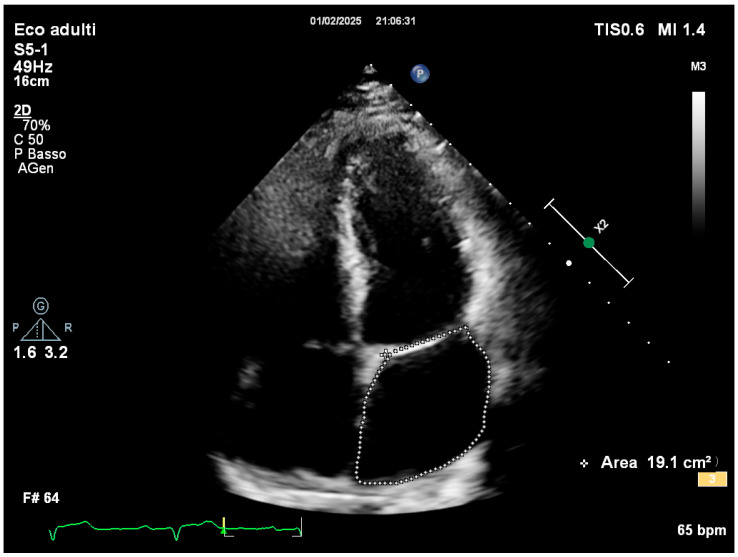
Left atrial area measured in apical 4-chamber view.
